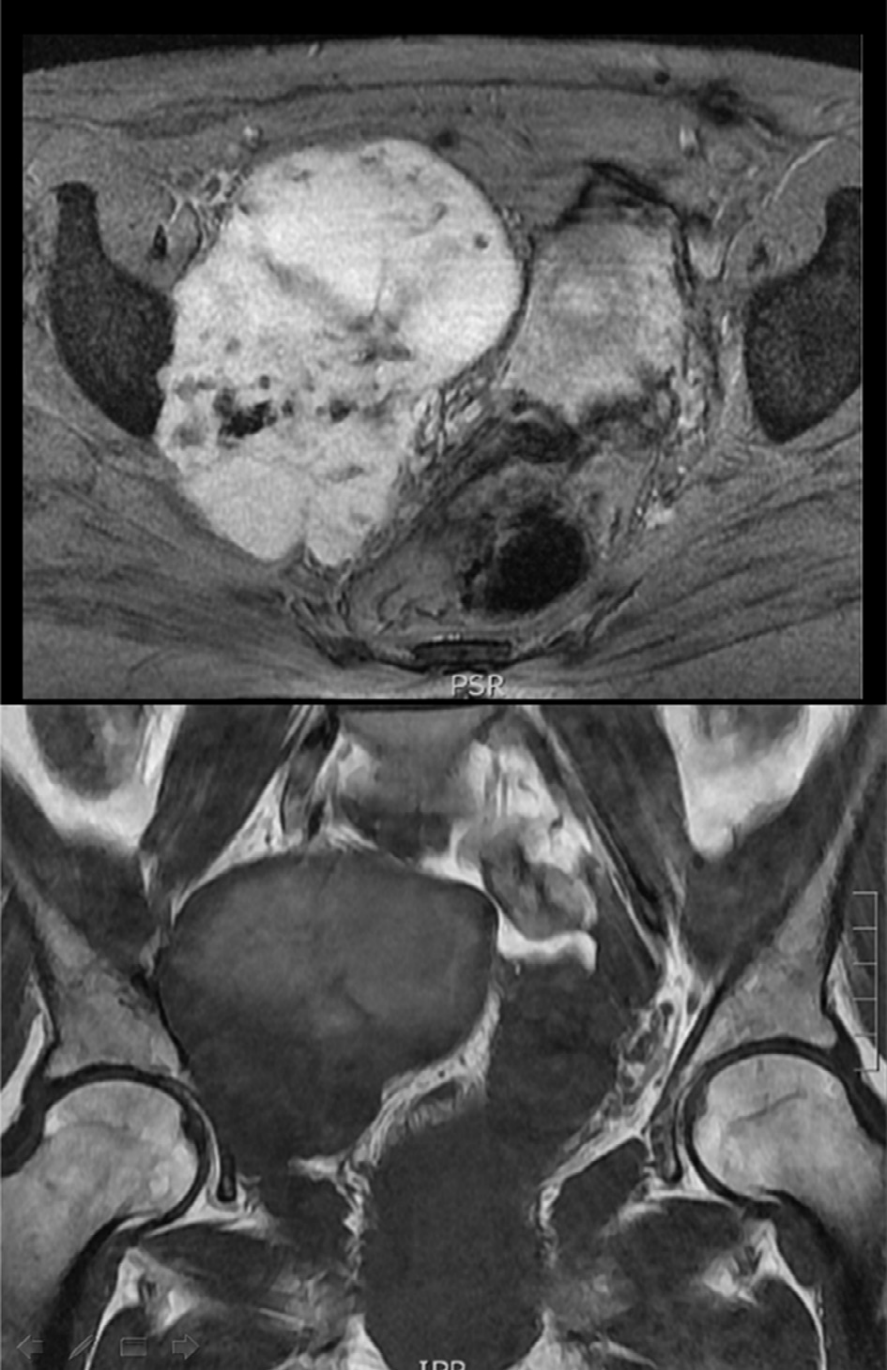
Magnetic resonance imaging (MRI) showing a polylobulated mass of soft tissue (11.2 × 7.6 × 9.1 cm) in the right obturator foramen affecting the internal obturator muscle with erosion of the acetabulum.
